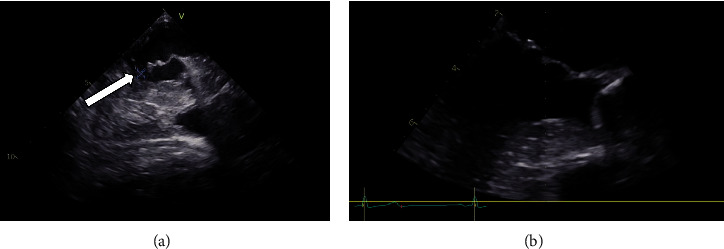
(a) Endocarditic lesion (4 × 5 mm) on the anterior mitral valve leaflet in the transesophageal view and (b) transesophageal echocardiographic control 8 weeks after diagnosis of endocarditis without detection of vegetation on the mitral valve.
